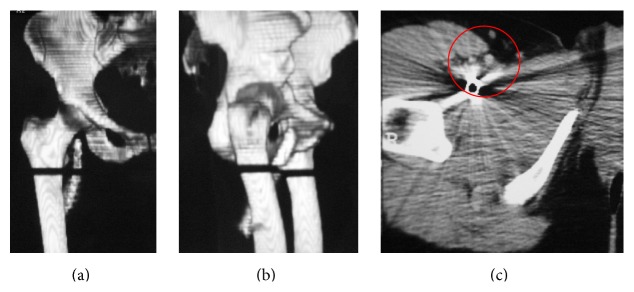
(a-b) CT-scan reconstruction. (c) Rod closeness to the femoral vessels.
